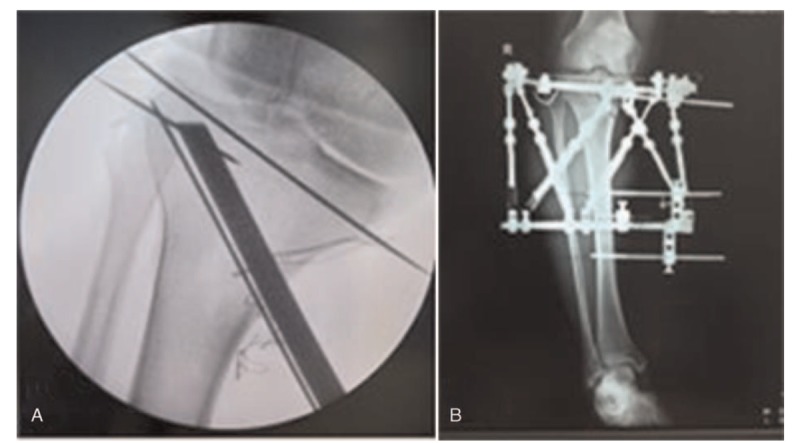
(A) X-ray of osteotomy position in HTO. (B) X-ray after installing external fixator in HTO operation. HTO = high tibial osteotomy.
